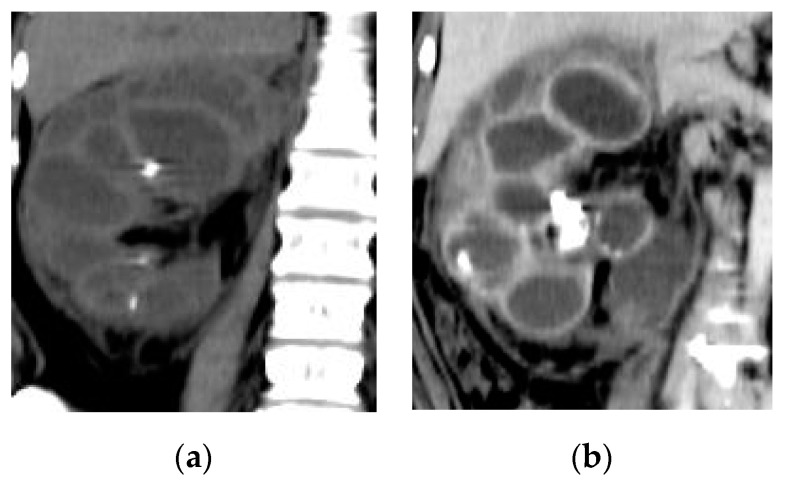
Right XGP. (a) Non-contrast and (b) post-contrast coronal reformatted CT images show stones at the renal pelvis and middle calyx with multiple parenchymal cavities.
